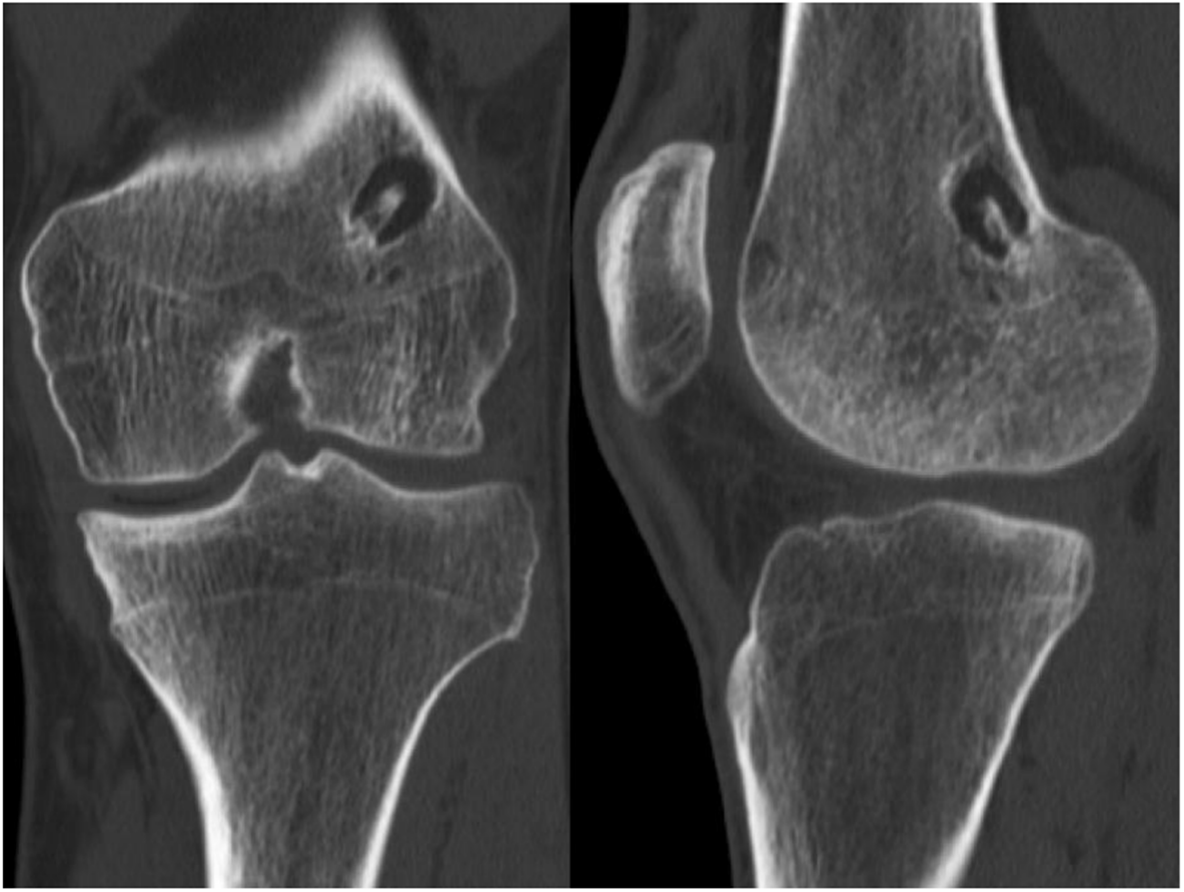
Frontal and sagittal view CT of a 20-year old patient with a re-rupture 2 years after ACL reconstruction. The outer femoral drill canal measures 11 mm
